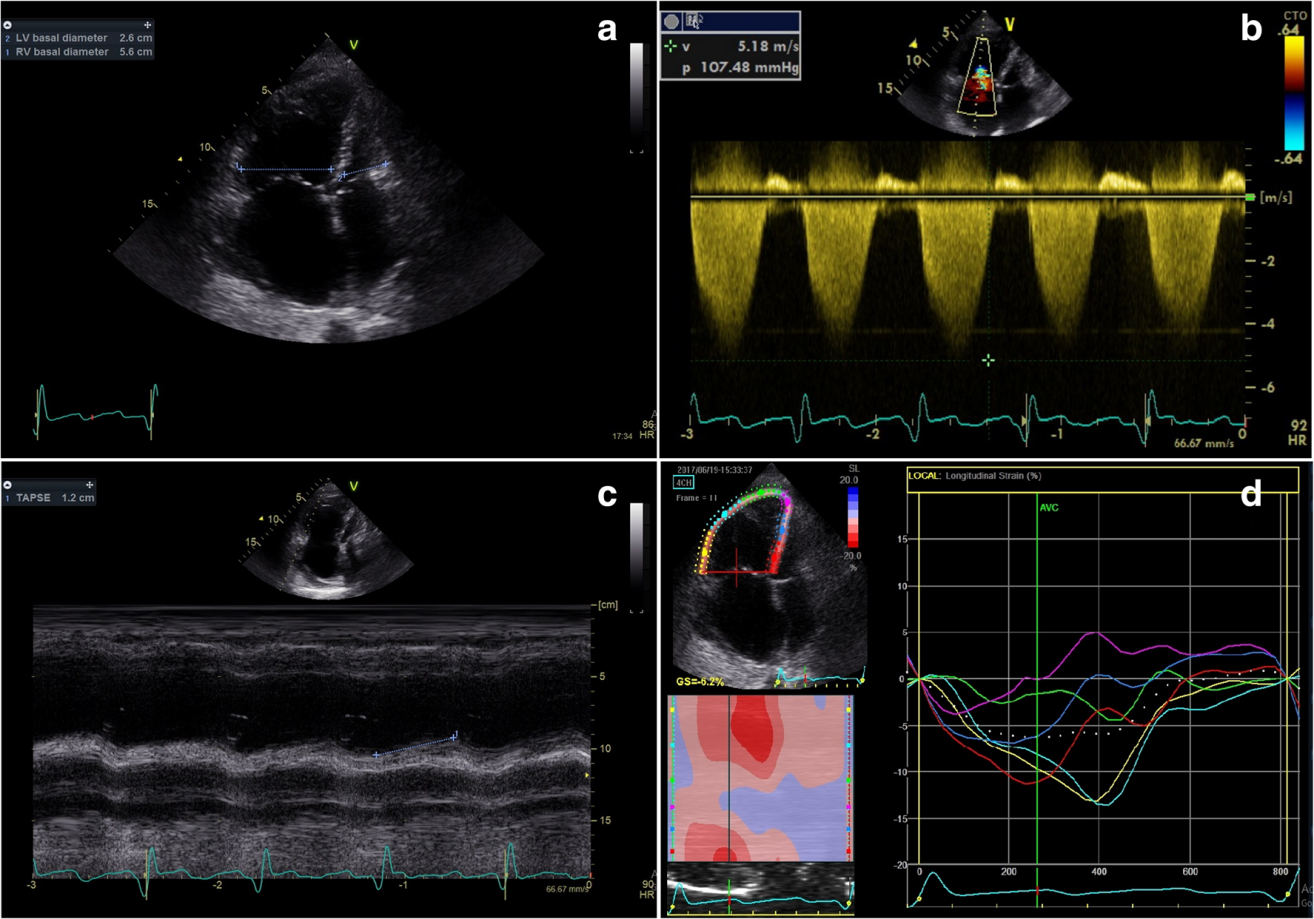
Relevant echocardiographic parameters found in chronic cor pulmonale. This figure shows the most common findings that can be found in the setting of chronic cor pulmonale. (a) Ratio between right ventricular (RV) and left ventricular (LV) basal diameters, often enough > 1, meaning a RV dilatation. (b) High right atrio-ventricular gradient, typical of chronic pulmonary hypertension. (c) Reduced tricuspid annular plane systolic excursion (TAPSE), reflecting a reduced RV longitudinal function. (d) Reduced RV longitudinal strain
